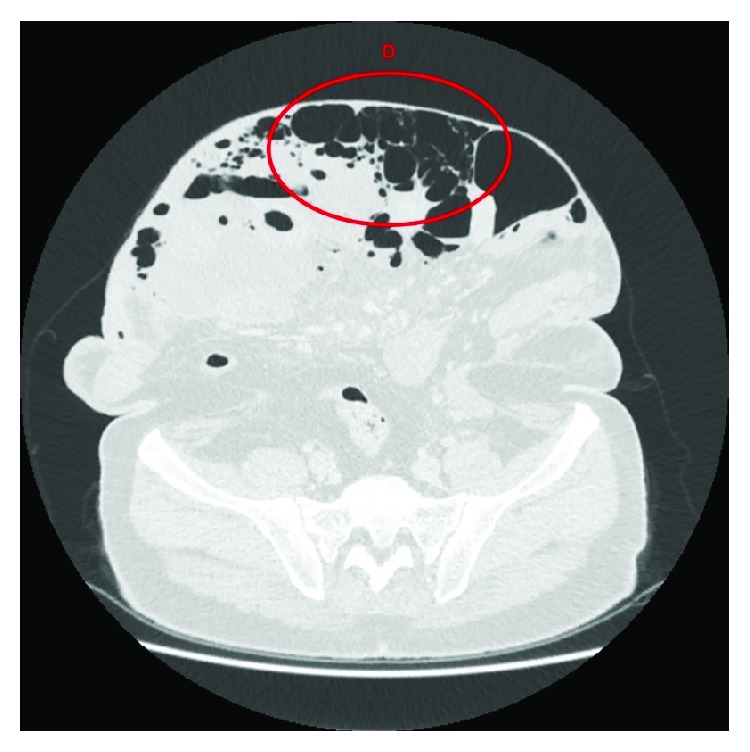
Axial CT image showing (D) septated free gas, presumed to be located in the mesentery of the colon.
